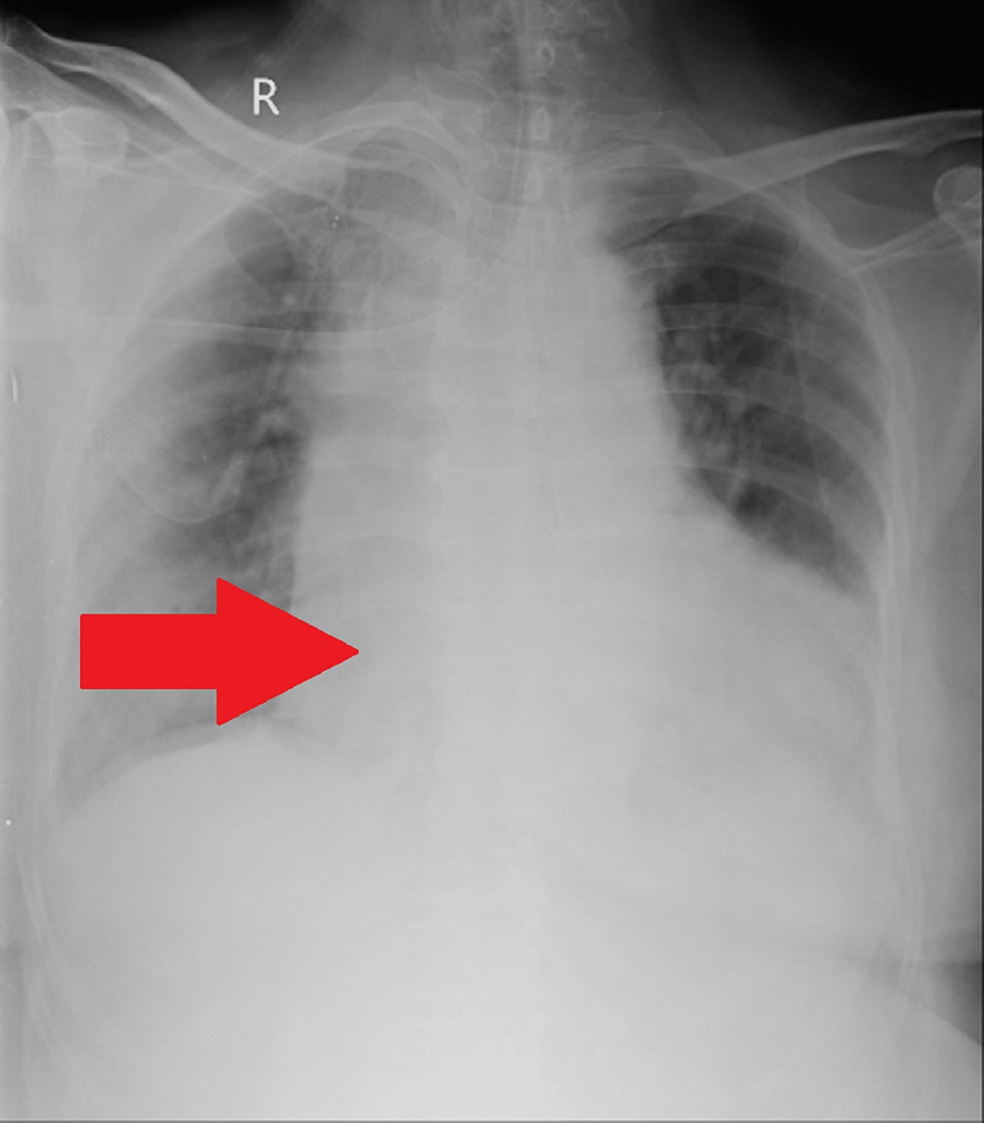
Chest X-ray Anterior-Posterior (AP) View - Demonstrate Massive Left Ventricular Hypertrophy. Widening of Mediastinum with Globular Shape is Consistent with Pericardial Effusion
